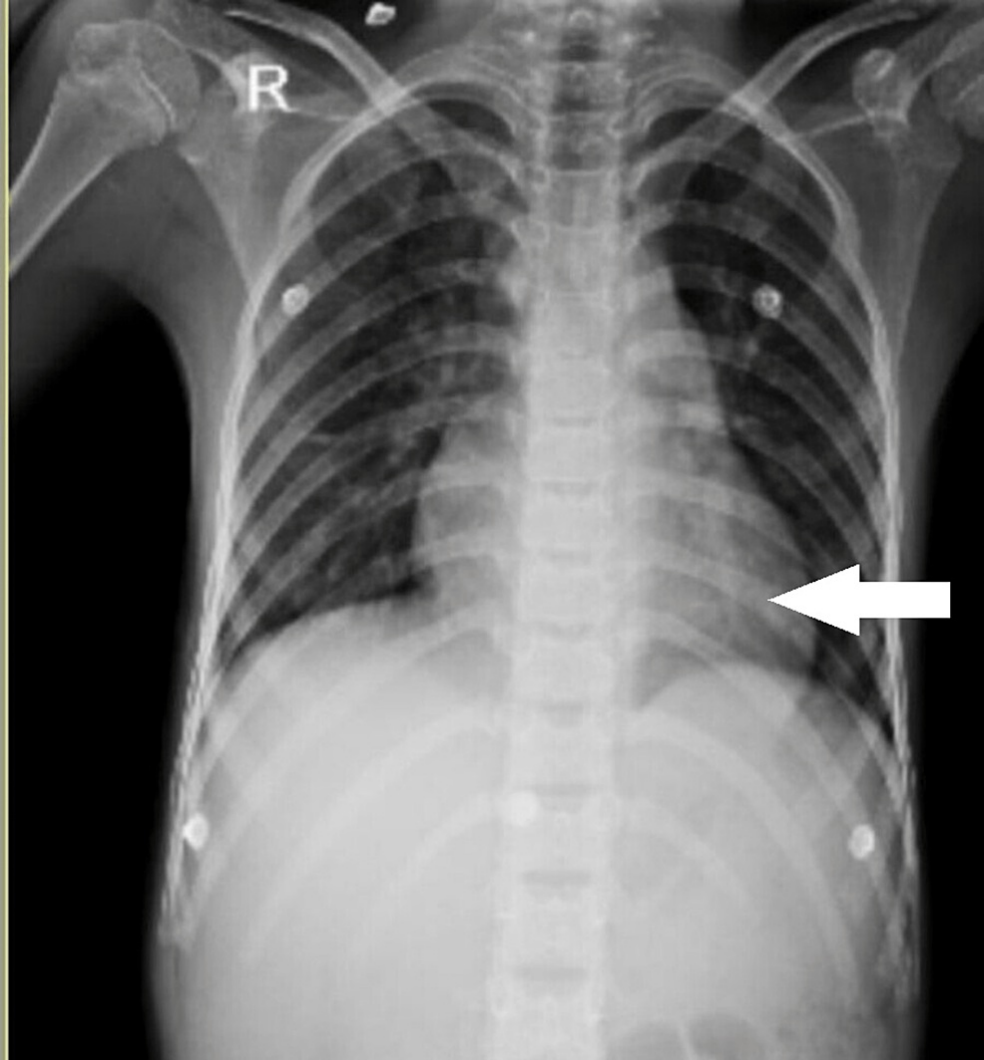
Chest X-ray of the patient revealed cardiomegaly
